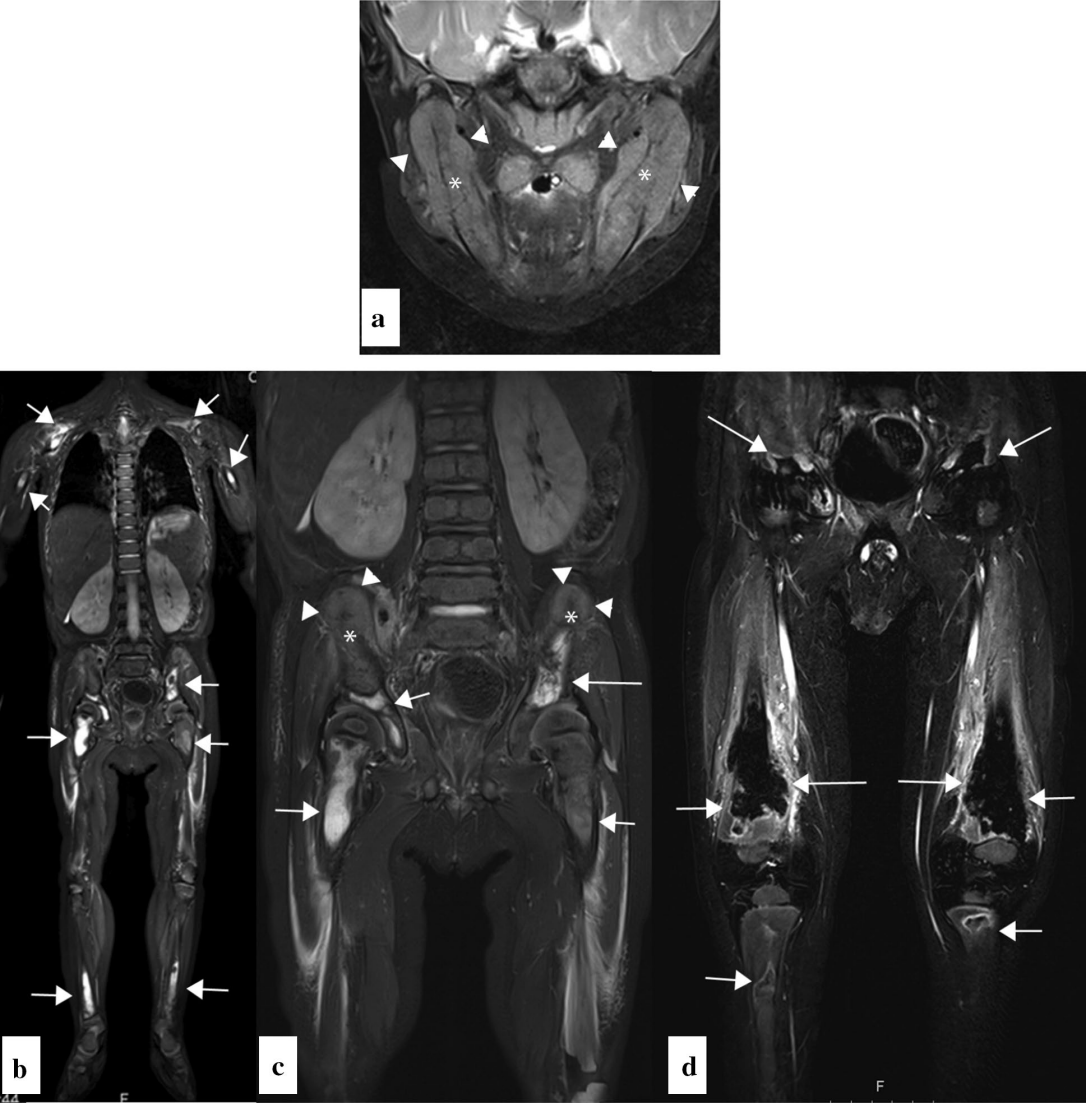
Coronal water-sensitive, fat-suppressed MRI-sequences of the mandible (a), whole-body (b), pelvis, and femurs of the AML patient (c, d) showing extensive infarcts in several bones (*arrows*). In the mandible and iliac wings, there was marked, spiculated cortical reaction and new bone formation (*arrowheads*) with destruction of the underlying skeleton (*asterisk*). Subtracted post contrast T1 fat suppressed images of the pelvis and femurs showed low-signal avascular areas with a peripheral rim of enhancement caused by the multiple infarcts (*arrows*). There was soft tissue edema in the lower extremities. The kidneys were slightly enlarged
